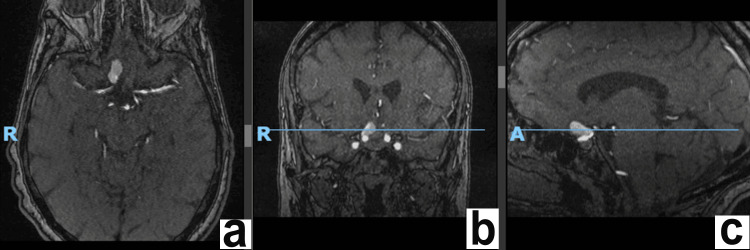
Preoperative axial (a), coronal (b), and sagittal (c) cuts of MRI angiogram. Demonstrating a large right paraophthalmic segment ICA aneurysm (measuring up to 17 mm), which arises at or just distal to the ophthalmic artery takeoff. This projects anterosuperiorly along the planum sphenoidale. ICA: internal carotid artery.
